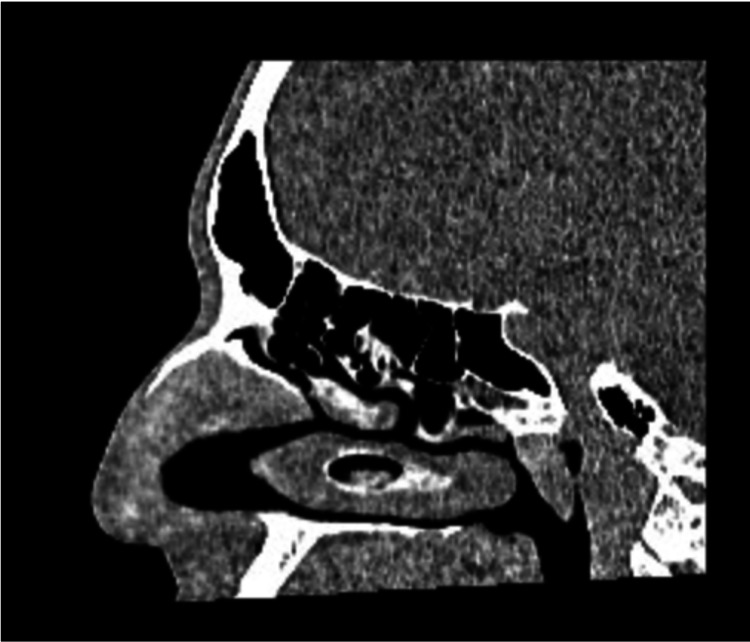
Normal anatomy revealed on CT scan of the affected area CT, computed tomography.
